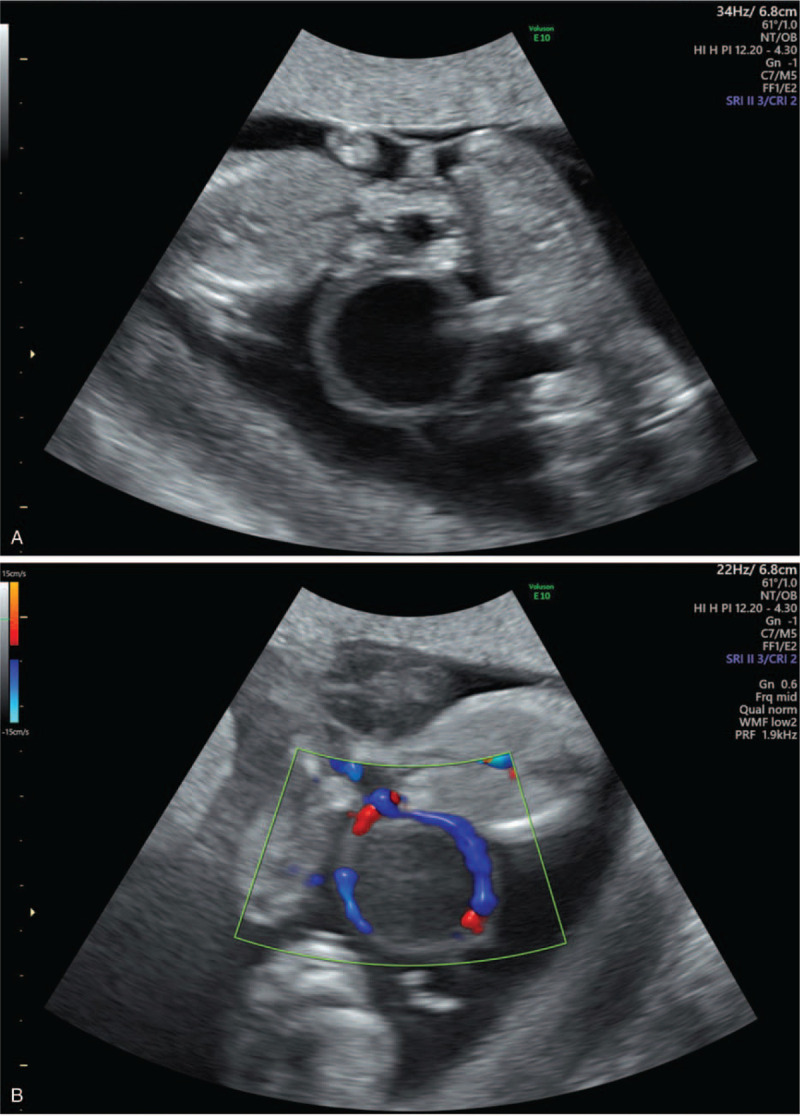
Two-dimensional ultrasound image (A) and color Doppler flow imaging (B) of the conjoined twins at 12 wk of gestation. The conjoined twins fused through the lower abdomen with a cystic structure.
